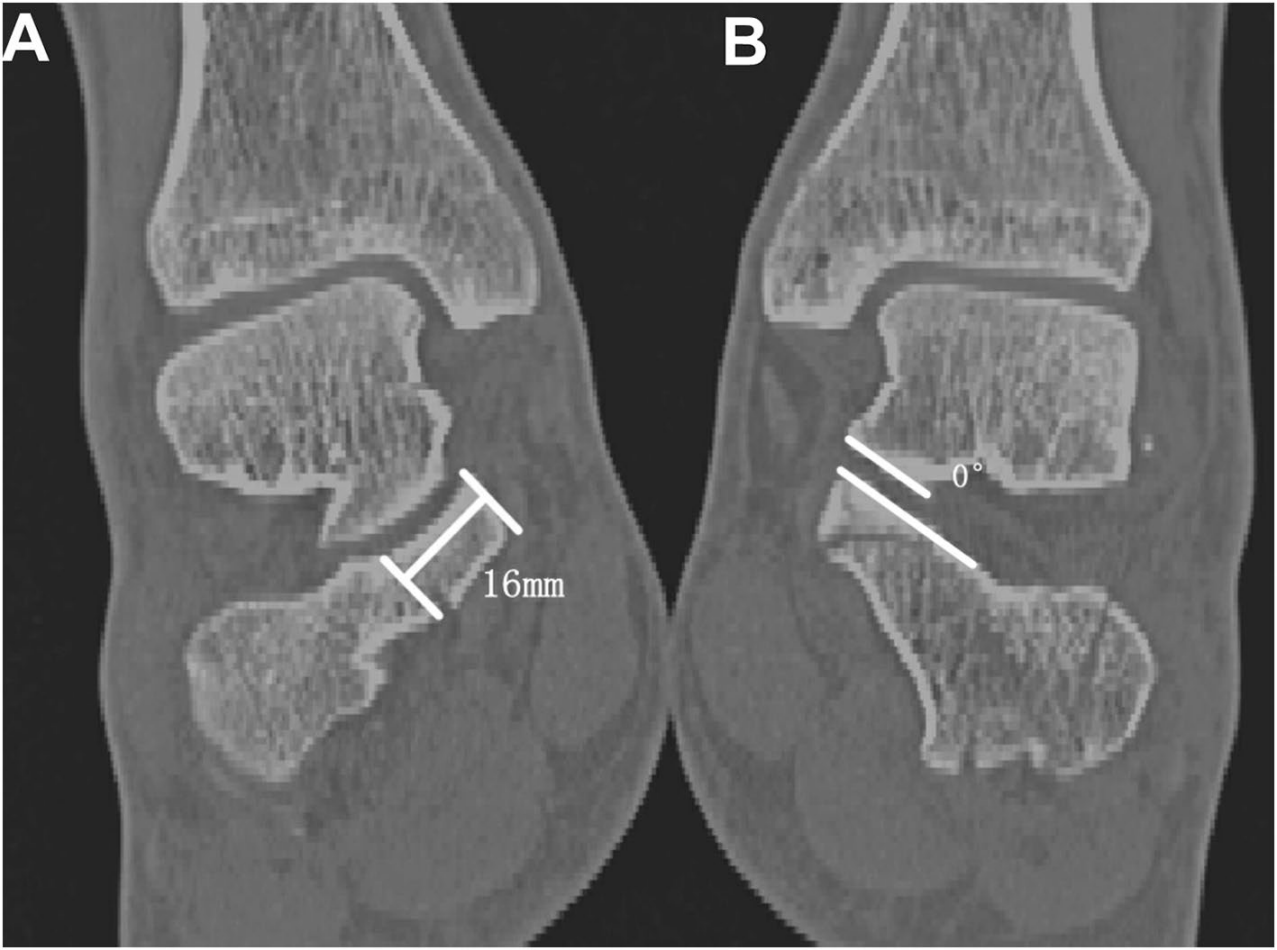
The method used for 2-D CT mapping of sustentacular fracture. (A) Coronal CT image showing the sustentacular fracture. (B) Assessment of angulation in the coronal.
